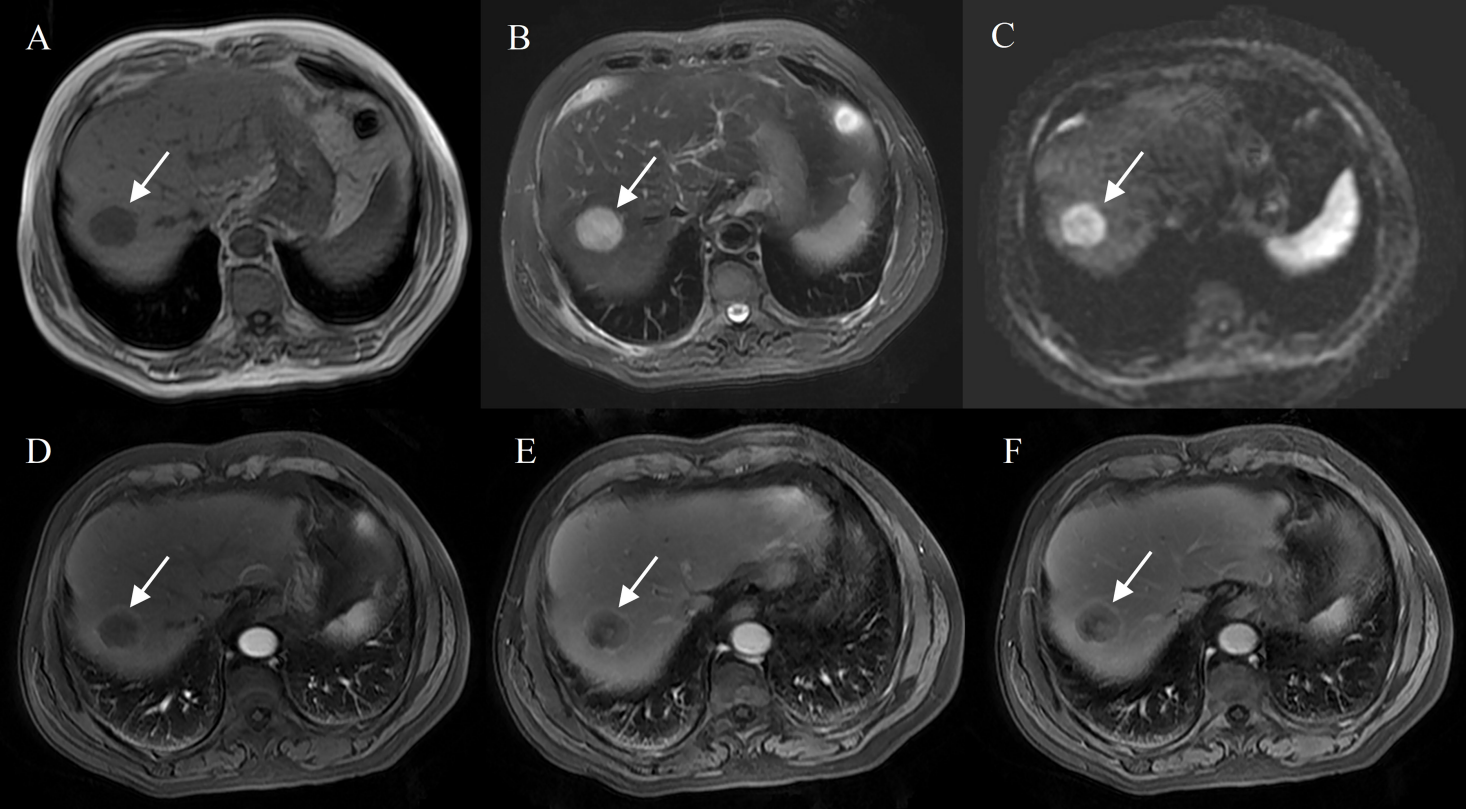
Hepatocellular carcinoma with peripheral rim enhancement in a 70-year-old male with hepatitis B virus. Gd-DTPA-enhanced MRI detected a round and smooth tumor (4.8 cm) with heterogeneous SI on T1WI and T2WI (A, B), restricted diffusion (C). Peripheral rim-like enhancement in the arterial phase (D), complete capsule enhancement on portal venous phase (E) and delayed phase images (F).
